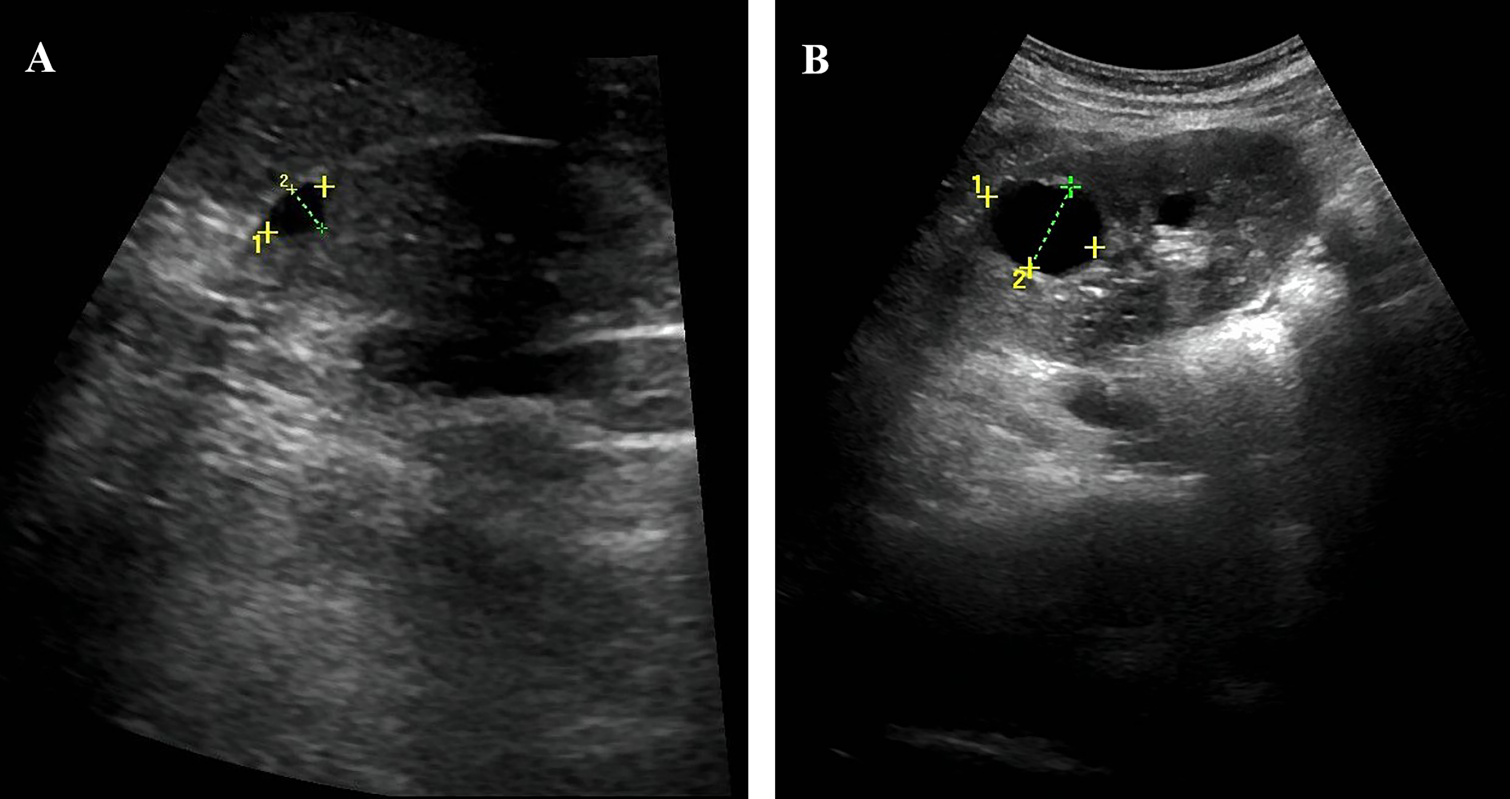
Abdominal ultrasound showed the patient’s kidneys. (A) The patient has multiple cysts in the right kidney, the largest cyst (9*6mm). (B) The patient has multiple cysts in the left kidney, the largest cyst (25*19mm).
